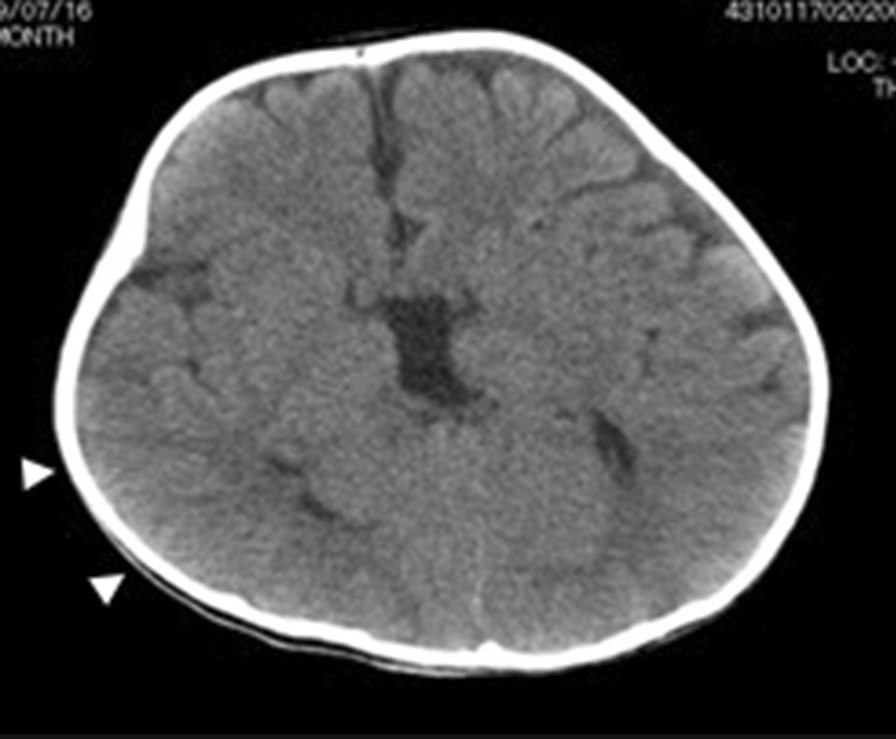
Computed tomography of the brain on postoperative day 1 showing localized cerebral edema with a narrowed sulcus in the right frontal and temporal lobes (white arrow head)
